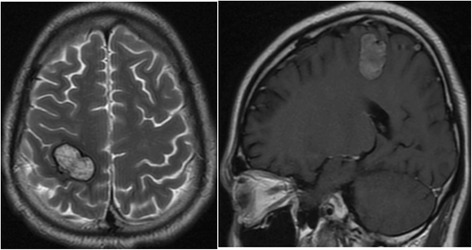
CT showing the decrease in size of right parietal lobe lesions in comparison with Fig. 16
